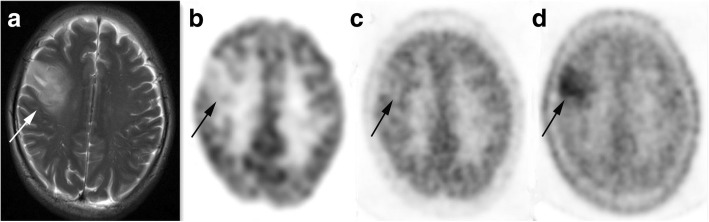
A patient aged 30–35 years with grade II glioma. T2-weighted MRI (a) shows a high signal lesion in the right frontal lobe (*arrow*). Higher uptake of ^11^C-MET (d), nearly equal uptake of ^13^N-NH_3_ (c) and apparently reduced uptake of ^18^F-FDG (b) were observed
